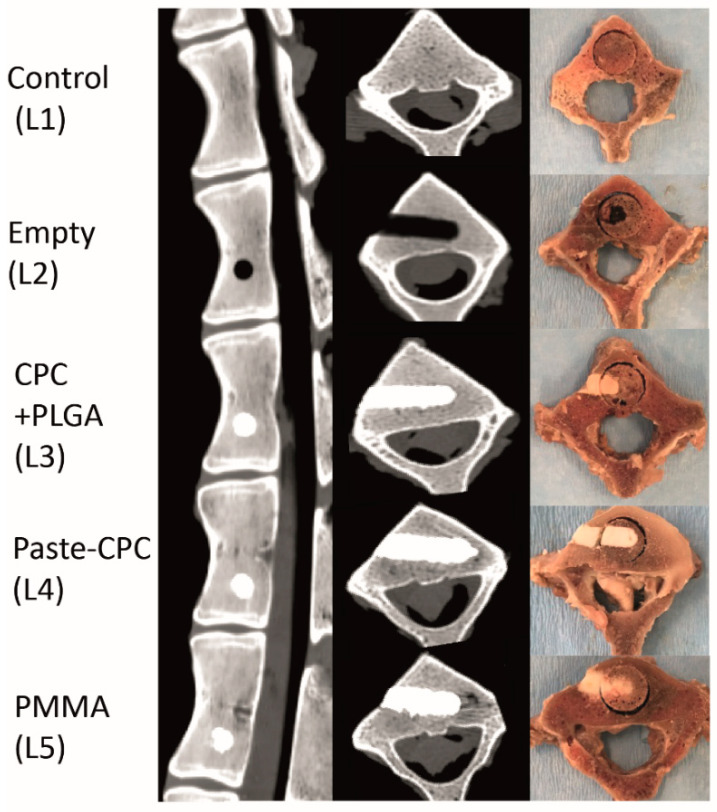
Depiction of cement localization by computed tomography (left—sagittal; middle—transversal) or gross morphology (right); L1 = untouched control; L2 = empty defect; L3 = CPC + PLGA; L4 = Paste-CPC; L5 = PMMA (Kyphon HV-R). CPC, calcium phosphate cement; PMMA, polymethylmethacrylate; PLGA, poly(L-lactide-co-glycolide). For scalebars please refer to Figure 2.
